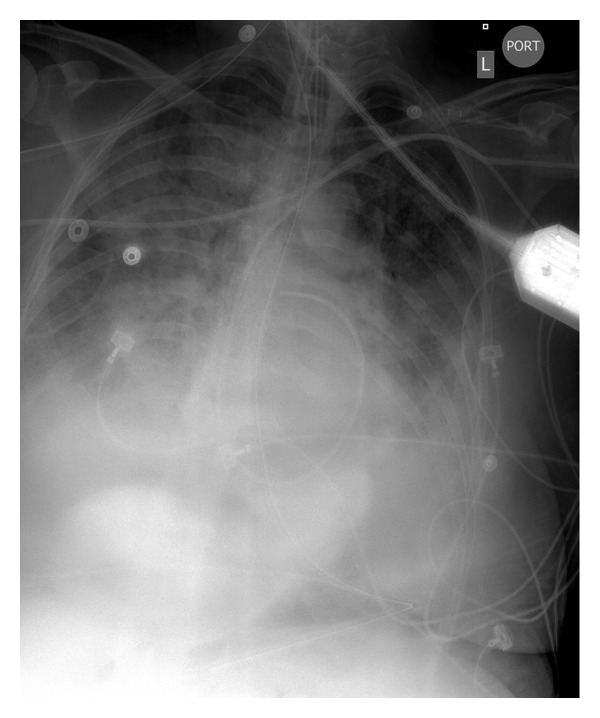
Chest X-ray of an ARDS victim that suffered a grade 4 SAH.
